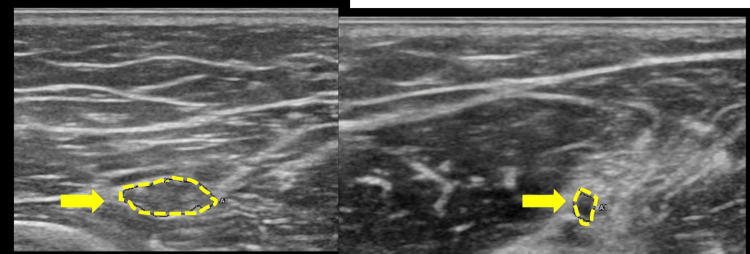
US showing radial nerve (yellow arrows). The radial nerve is enlarged (left side) and its proximal side is narrowed (right side). There is no obvious space-occupying lesion. US, ultrasonography
